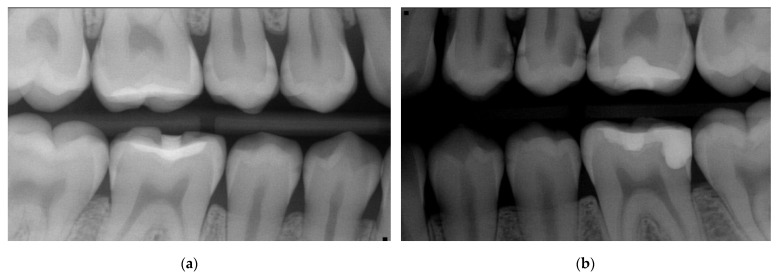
Case 2—Bitewings (03/2023) on the right (a) and left side (b) at the 1-year follow-up after SF application. The patient’s age is almost 17. The radiographic situation shows both the progression (only in second quadrant (b)) and stagnation of (both enamel and D1) proximal lesions after single application of SF within regular recall visits.
